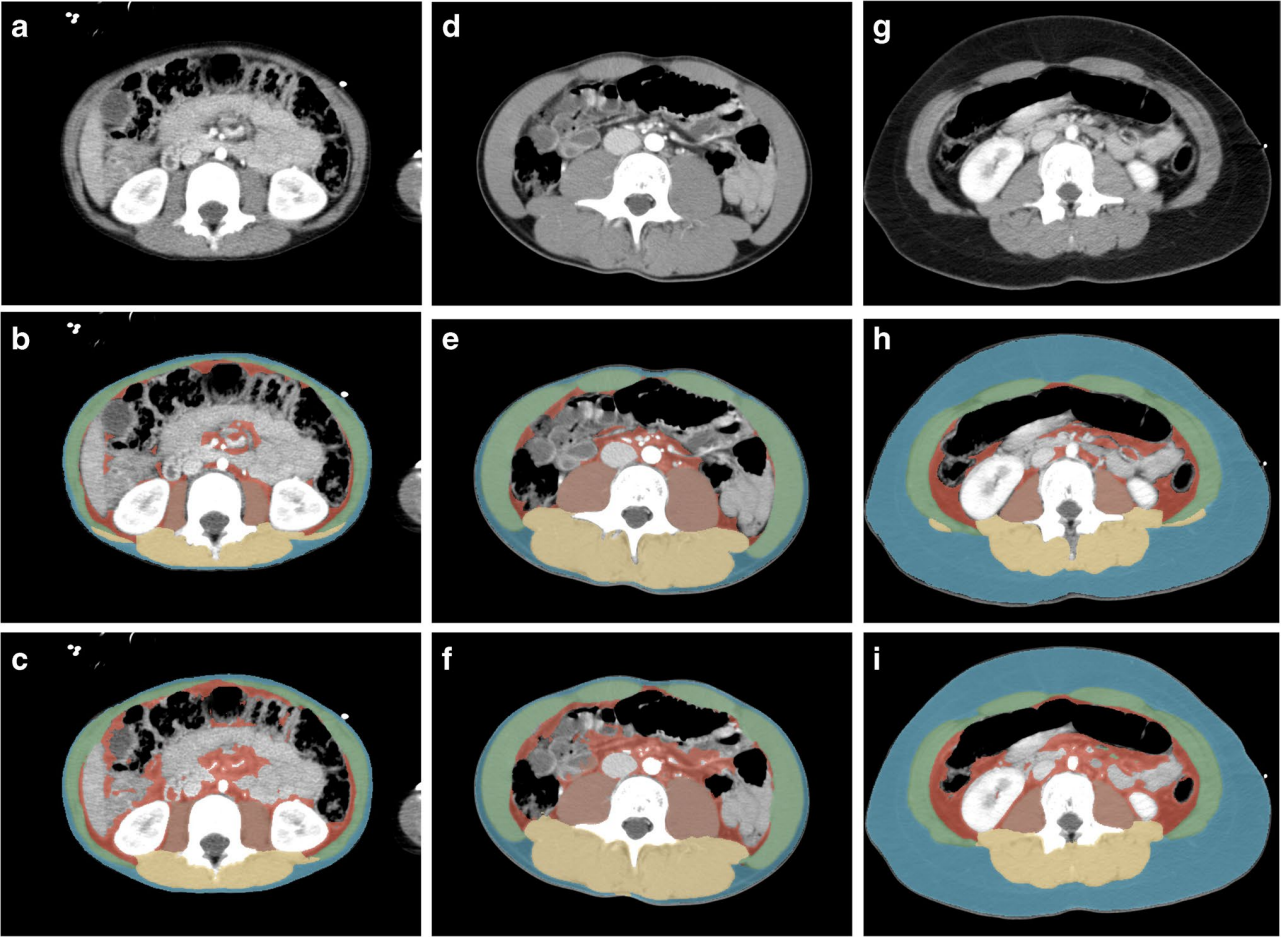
Axial computed tomography images obtained in a 5-year-old boy (a–c), a 15-year-old boy (d–f) and a 12-year-old girl (g–i) at the level of the third lumbar vertebral body performed following high-energy trauma. Contrast-enhanced images before segmentation (a, d, g) and after manual (b, e, h) and automatic (c, f, i) segmentation. Automatic segmentation sometimes included parts of the intestines as visceral fat and often failed to differentiate the abdominal wall muscles from the latissimus dorsi muscles. *Blue* subcutaneous fat, *green* abdominal wall muscles, *red* visceral fat, *orange* psoas muscles, *yellow* paraspinal muscles
